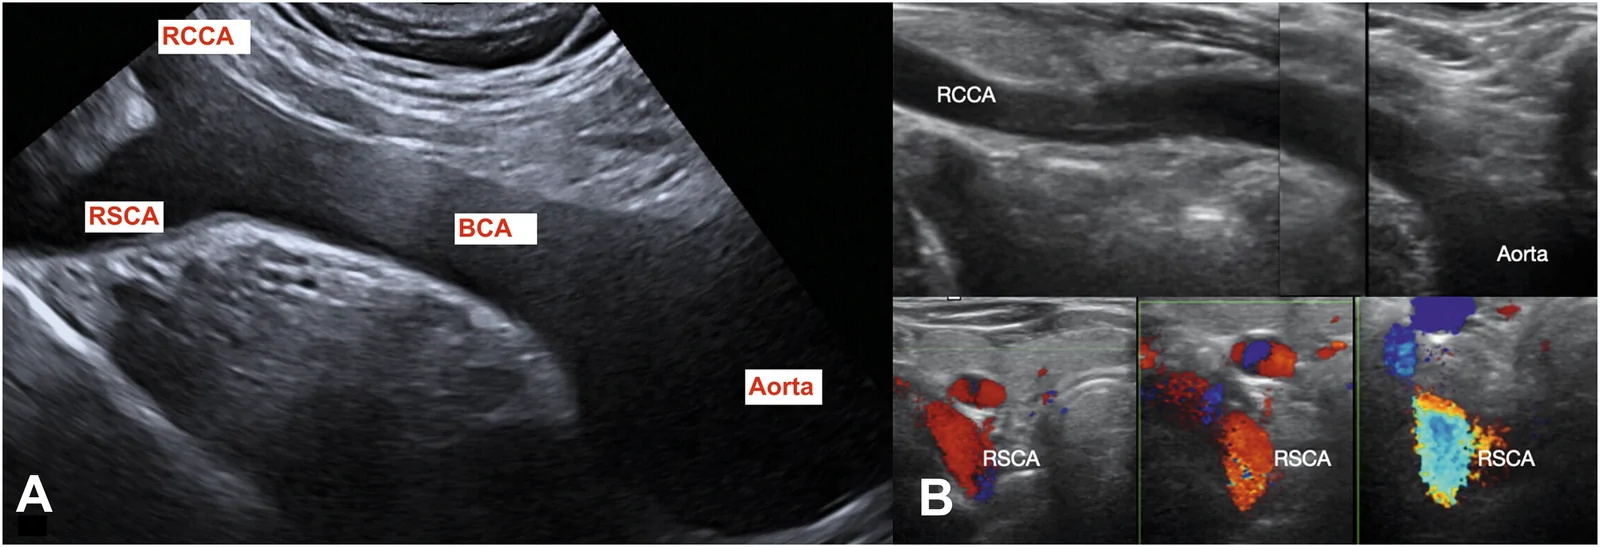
Sonographic patterns of brachiocephalic artery origin. (A) Normal “Y-image” bifurcation. (B) Anatomical variant: separate origins of the right common carotid artery (RCCA) and right subclavian artery (RSCA) from the aortic arch. Reproduced with permission (25).
